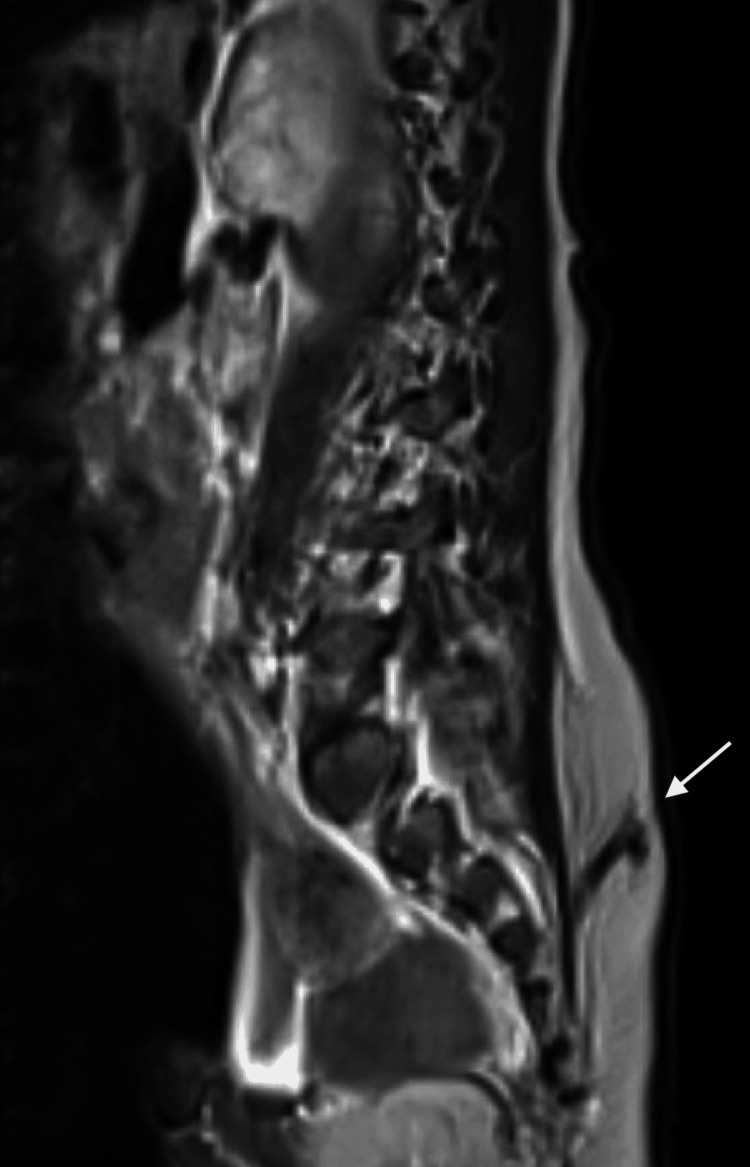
T1-weighted fluid-attenuated inversion recovery spinal MRI (sagittal view) demonstrating sacral dermal sinus tract (white arrow) The sacral dermal sinus tract served as the definitive diagnostic finding to establish a communication between the skin and spinal canal, explaining the patient’s recurrent meningitis.
